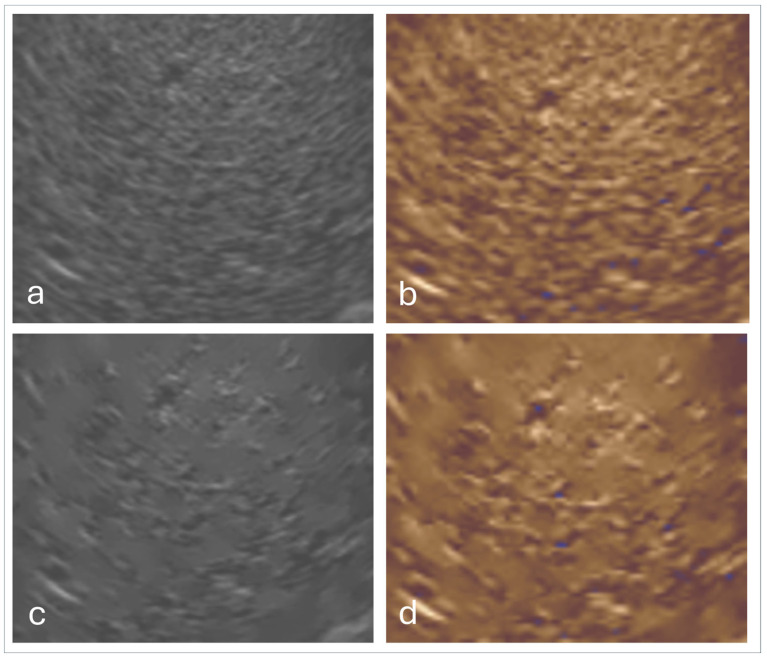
Sonographic liver texture in a patient with chronic hepatitis B visualized with standard greyscale imaging (a). Photopic imaging (Sepia) leads to significantly more vivid detail recognition (b). The remodeling signs of hepatitis, highlighted with a speckle algorithm (c), are also better visualized after Photopic (d).
